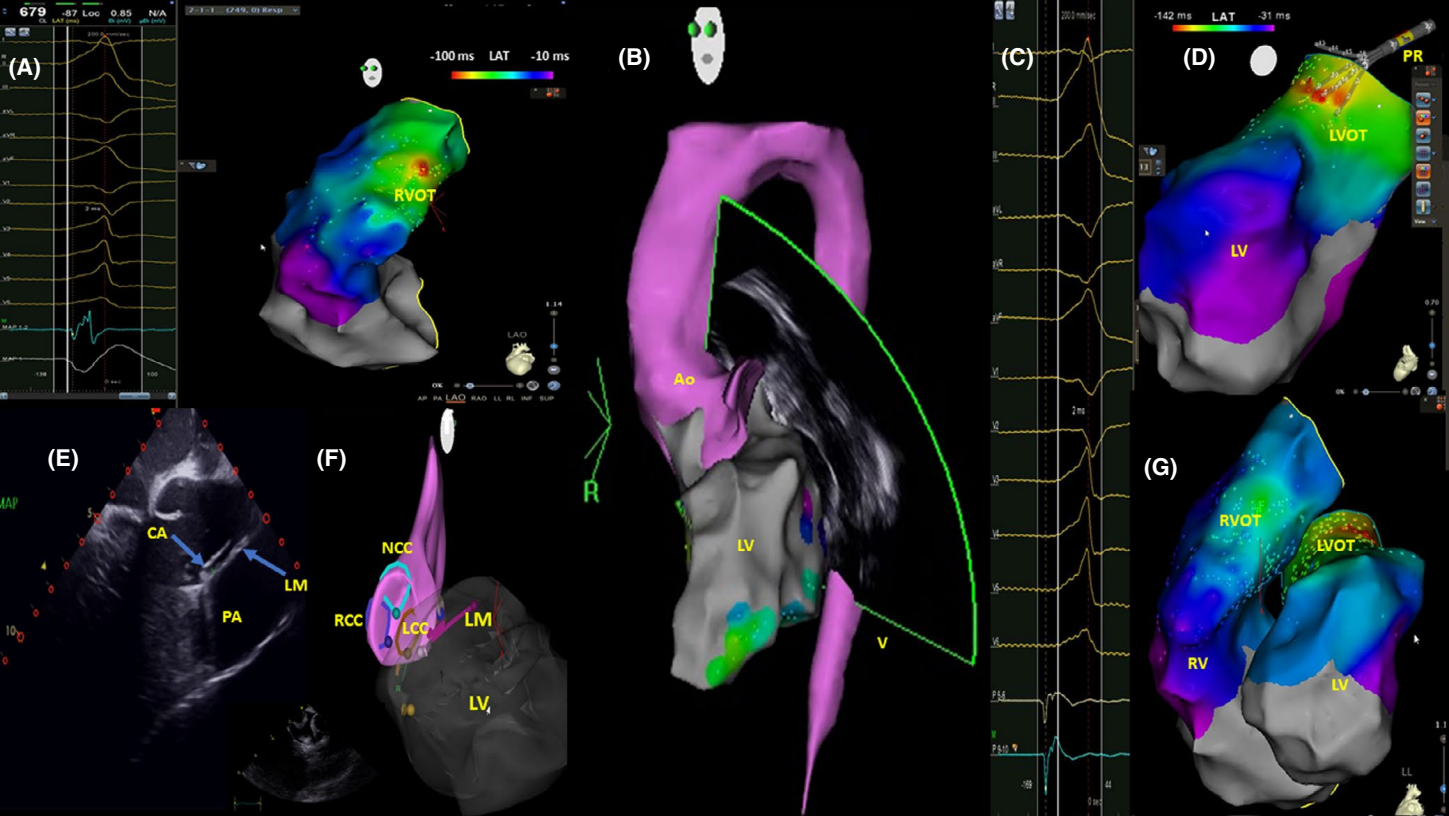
A, EGM and LAT during RVOT mapping. B, The anatomical Carto‐map that showing left ventricle and aorta. C, EGM stored using pentaRay catheter during LVOT mapping. D, LAT during LVOT mapping. E, 2D intracardiac echocardiography. The tip of the ablation catheter was positioned near left main coronary artery. F, ICE map with LCC and LM, RCC, NCC, and LV merged with anatomical map of Aorta and left ventricle; G, LAT of left and right ventricles; the earliest point of activation shown in the column was registered in LVOT, at left coronary cusp, near left main coronary artery. Ao, aorta; CA, tip of ablation catheter; EGM, electrograms; ICE, intracardiac echocardiography; LAT, local activation time; LCC, left coronary cusp; LM, left main coronary artery; LV, left ventricle; LVOT, left ventricle outflow tract; NCC, no coronary cusp; RCC, right coronary cusp; RVOT, right ventricle outflow tract
